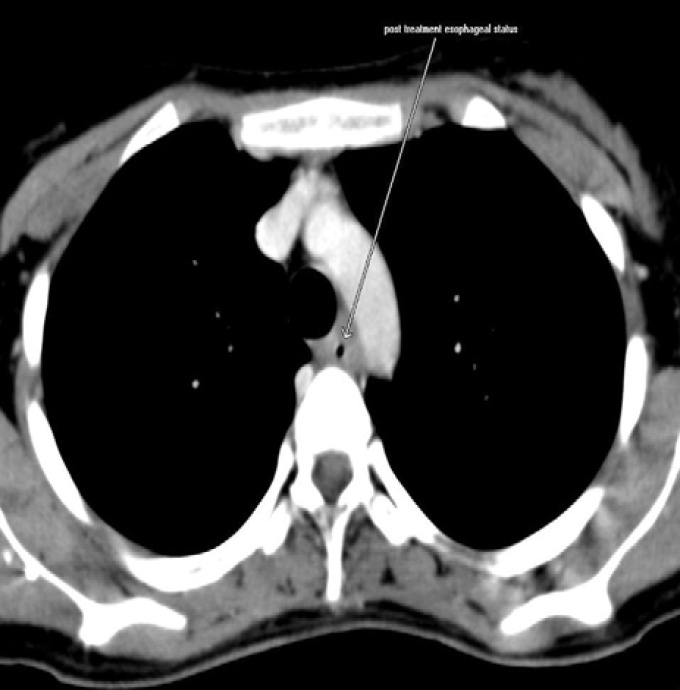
Post-treatment CT image shows complete disappearance of the left parasternal mass with normal esophageal thickening
